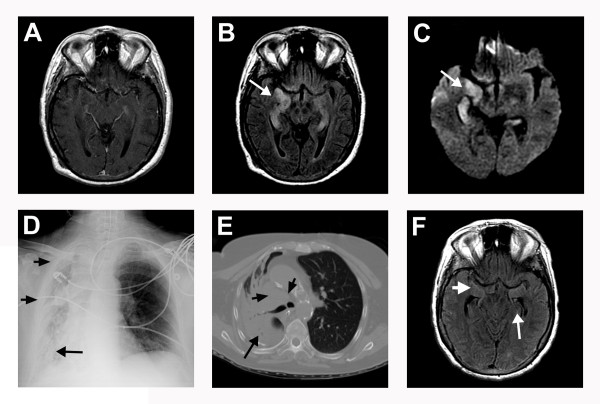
Magnetic resonance imaging (MRI) of the brain. (A) T1-post-gadolinium sequence with no enhancement. (B) T2-fluid-attenuated inversion recovery (FLAIR) sequence with hyperintensities in both mesial temporal lobes (arrow). (C) Diffusion-weighted images with T2 shine-through in the right temporal lobe and hippocampus (arrow). (D) Chest X-ray with right lower lobe infiltrates consistent with post-obstructive pneumonia (long arrow), complete opacity of the right middle and upper lobes due to atelectasis from small-cell lung cancer (short arrows). (E) Chest computed tomography scan with contrast of mediastinal and hilar lymphadenopathy (short arrows) and a loculated right lower lobe pleural effusion (long arrows). (F) MRI of the brain 6 months after initial neurological presentation with temporal horn enlargement (short arrow), hippocampal atrophy (long arrow) and resolution of T2-FLAIR hyperintensities.
